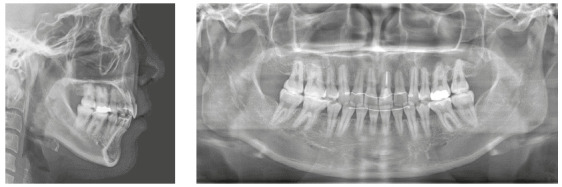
Two-year retention lateral cephalogram and panoramic radiograph.
